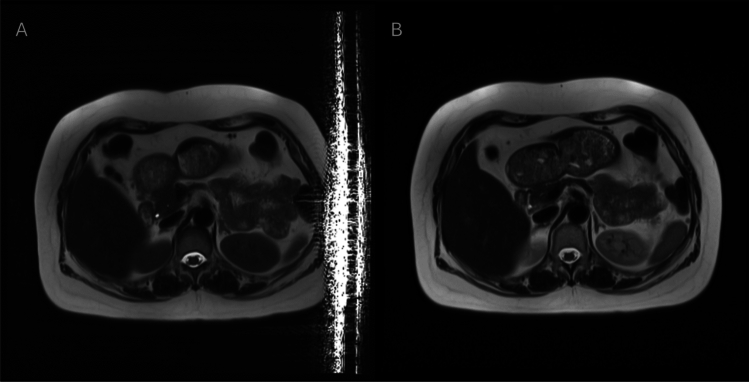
Abdominal T2 HASTE MR images in the same subject (A) with and (B) without external RF interference. In this example, the external RF interference appeared when MRI scanning was being performed on a neighbouring MRI system at the same nominal field strength (3 T). The MRI scanners were ramped up with a separation of around 100 kHz in their centre frequencies, a practice that is commonly recommended for neighbouring MRI systems at the same nominal field strength, but this was insufficient to avoid the presence of the RF interference on MRI sequences with high receiver bandwidths. The receiver bandwidth in these images was 725 Hz/pixel and with a matrix size of 384 in the frequency encode direction the resulting frequency range between the centre and edge of the image was 139.2 kHz. The RF attenuation at the location of the MR exam room door for each MR system were subsequently measured as 23 dB and 37 dB respectively. These low values were found to arise from missing/misaligned strips of RF leaves underneath the base of each of the doors.
